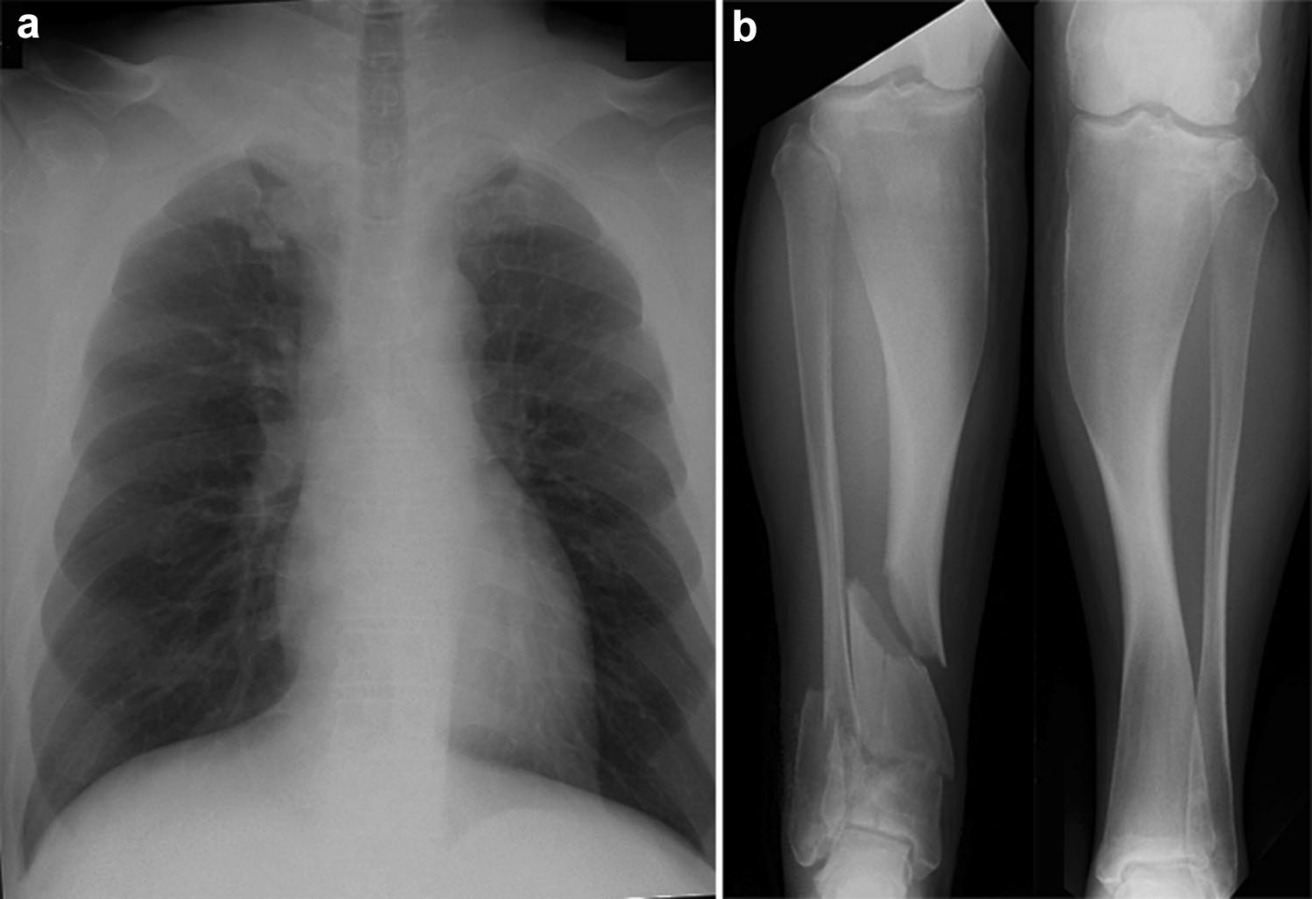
a, b An adult patient with metaphyseal dysplasia (Pyle disease). Radiographs show broad clavicles and ribs as well as significant expansion and thin cortices of the metaphysis and metadiaphyseal junction of the long bones giving rise to “Erlenmeyer flask” deformities. There are fractures of the right distal tibia and fibula
